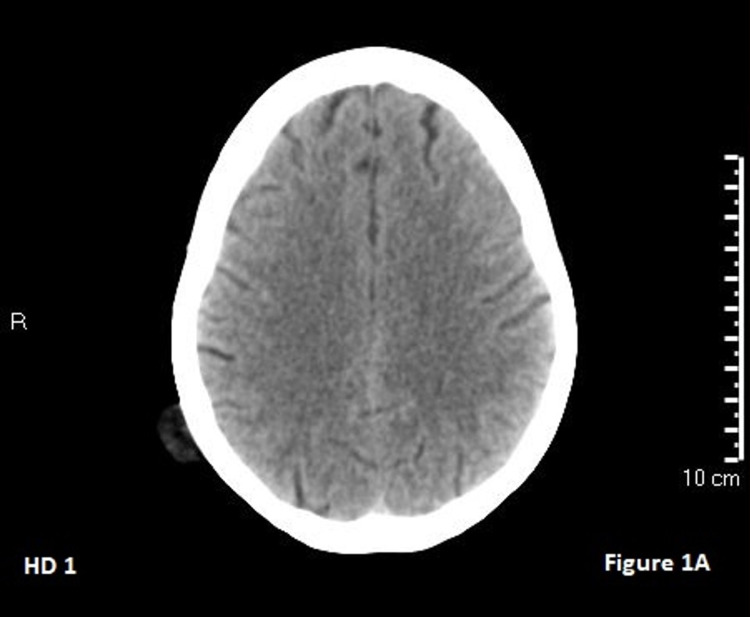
Hospital day 1 CT head shows no clear abnormalities.
